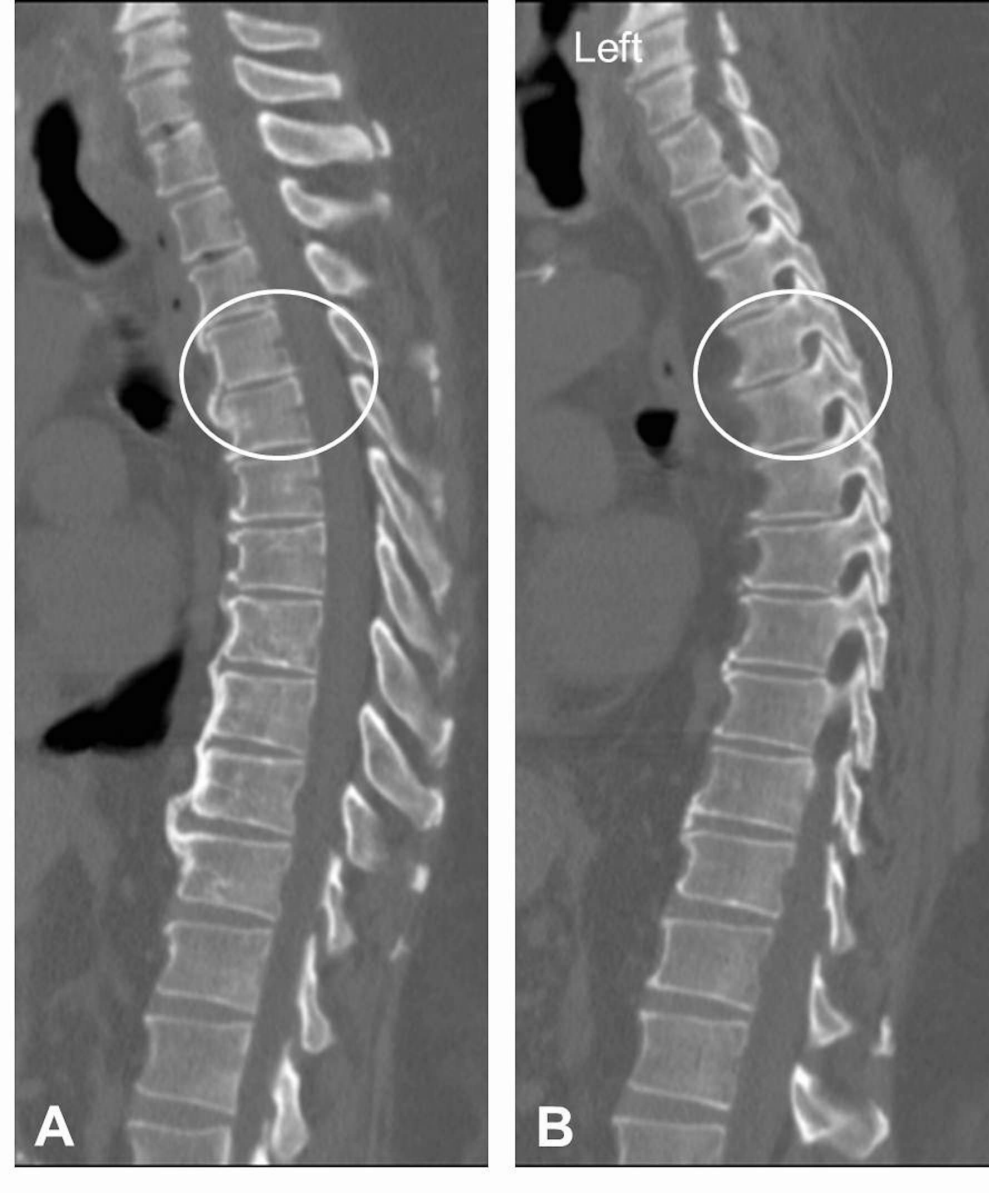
CT Following Recumbency Treatment A sagittal CT 16 weeks after recumbency treatment of the extension injury shows normal anatomic alignment of the thoracic spine as well as fracture healing with anterior osteophyte bridging between T4 and T5 (A, circle). A left parasagittal cut shows restoration of alignment and no distraction at the level of the facet joint (B, circle).
